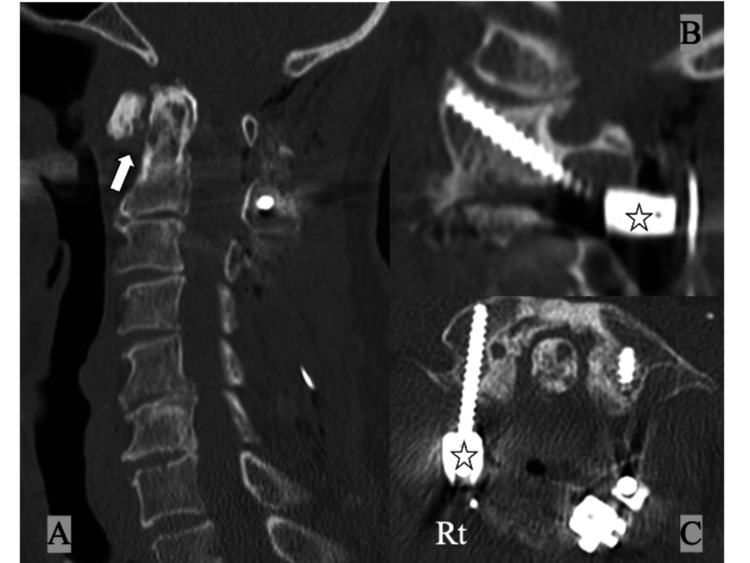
Postoperative CT of the cervical spine showing (A) good reduction of the atolantoaxial subluxation (white arrow), (B, C) the C1 lateral mass screw inserted from the caudal side of the right C2 nerve root (ponticulus posticus side, white stars).
